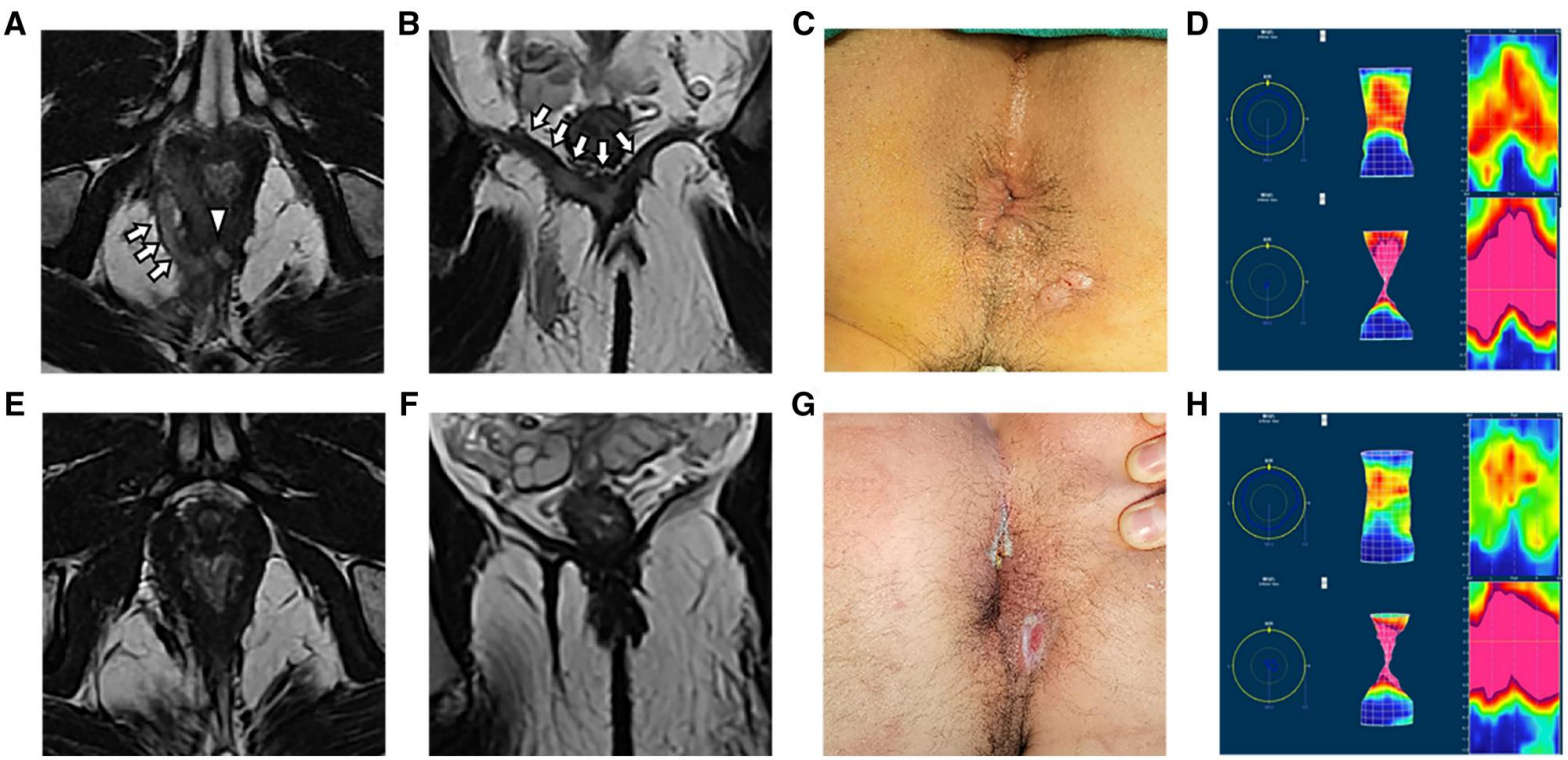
A high transsphincteric anal fistula with abscess in the right ischiorectal fossa and bilateral intra-levator ani muscle were treated by fistula occlusion with the internal sphincter flap with complete healing on follow-up. Preoperative and postoperative magnetic resonance imaging scans were performed to verify the complexity of the tract and internal opening and recovery by (A, E) axial T2-weighted images and (B, F) coronal T2-weighted images. (C, G) Photograph of the anus shown preoperatively and at the last follow-up. (D, H) The anorectal manometry assessments were carried out preoperatively and at the last follow-up. The arrows are pointing at the tracts. The triangle represents the IO. IO = internal opening.
